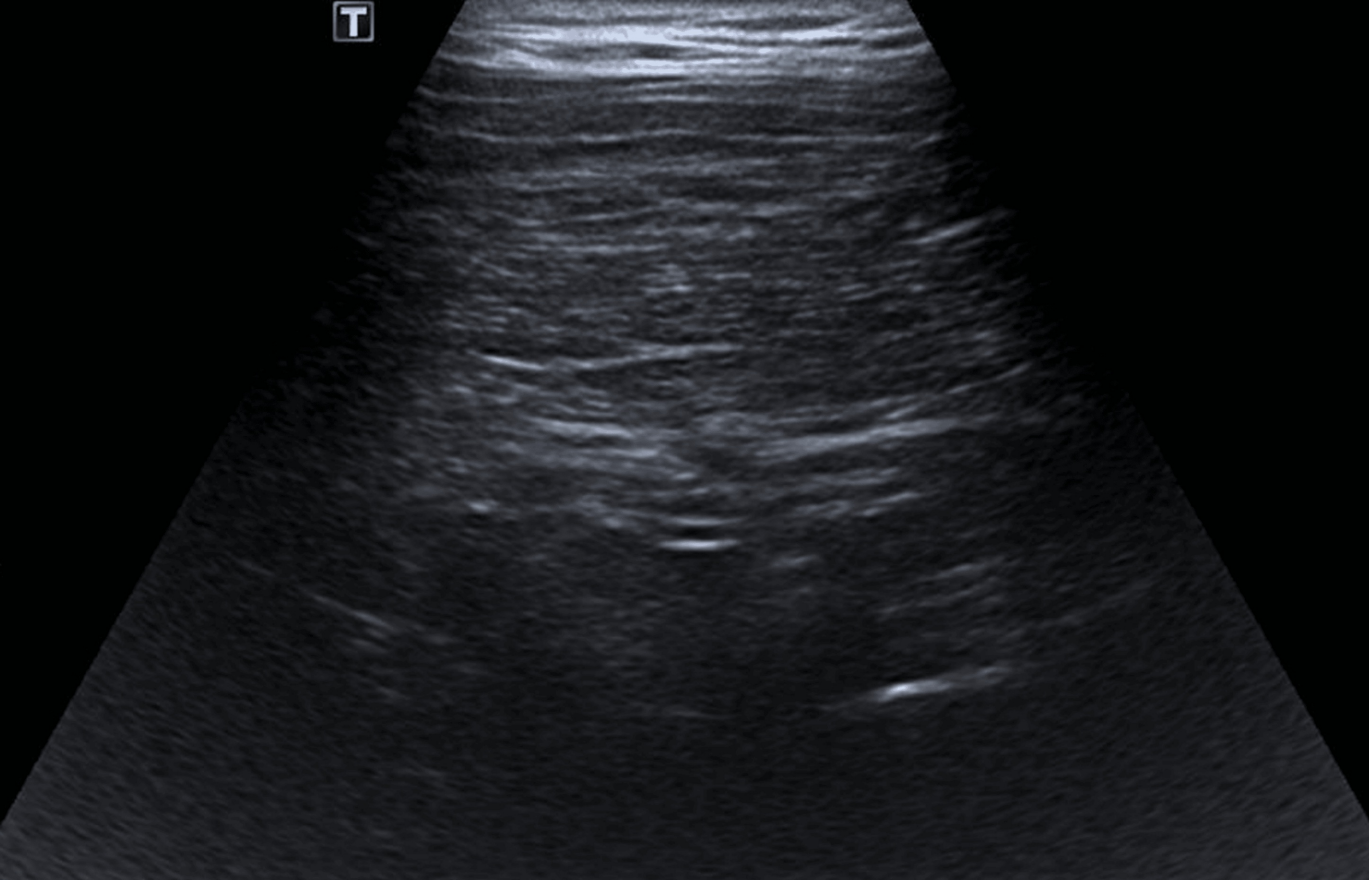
Ultrasound image of the left neck and upper back showing a large, encapsulated, iso- to mildly hyperechoic mass measuring over 11 cm. The mass is located approximately 1.1 cm from the skin, superficial to the left subclavian vein. It shows no posterior acoustic shadowing, internal vascularity, calcifications, or nodular soft tissue components.
